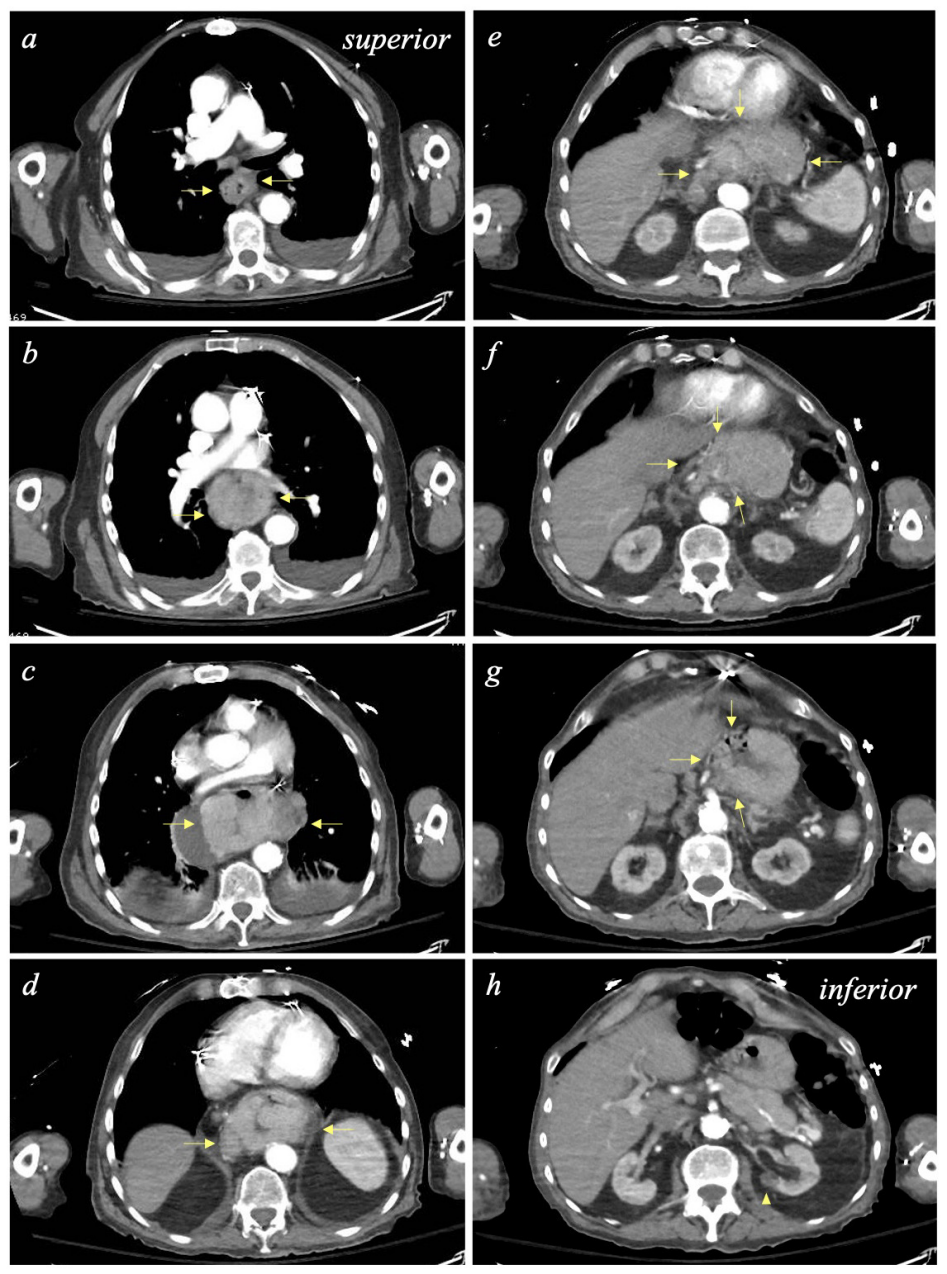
Computed tomography (CT) scans of the chest, abdomen, and pelvis with contrast reveals a large mass. [
